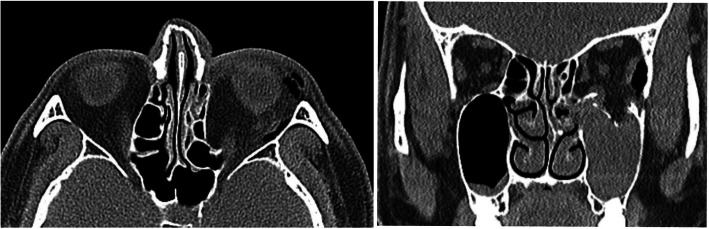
Preoperative CT (axial and coronal sections)
